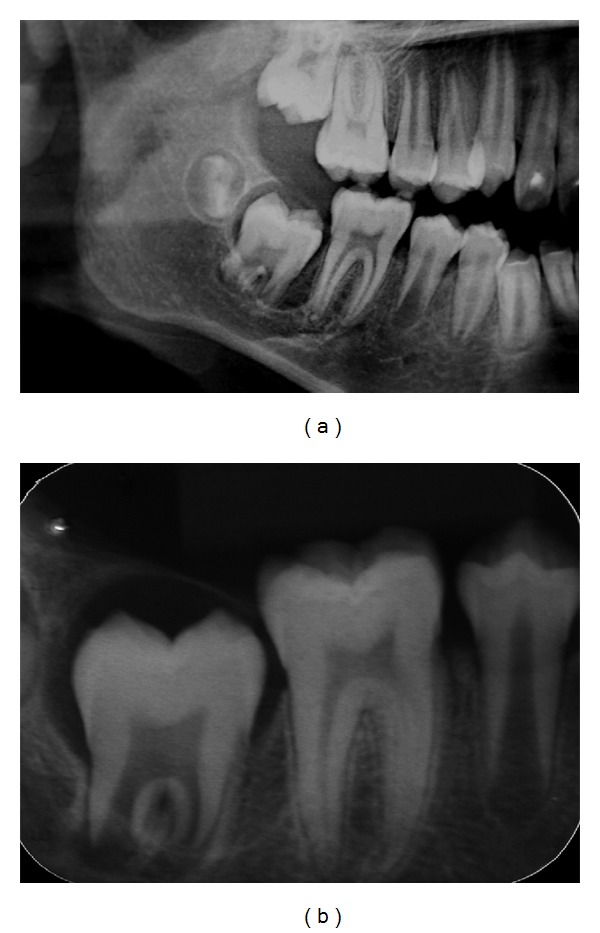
Radiographic aspect of the unerupted teeth. (a) Sectioned panoramic radiography: radiolucent well-defined area surrounding the crown of tooth 47, extending to apical region in the anterior area. (b) Periapical radiography: delicate sclerotic border, normal enamel and radicular formation, and absence of visible calcifications in pericoronal space.
